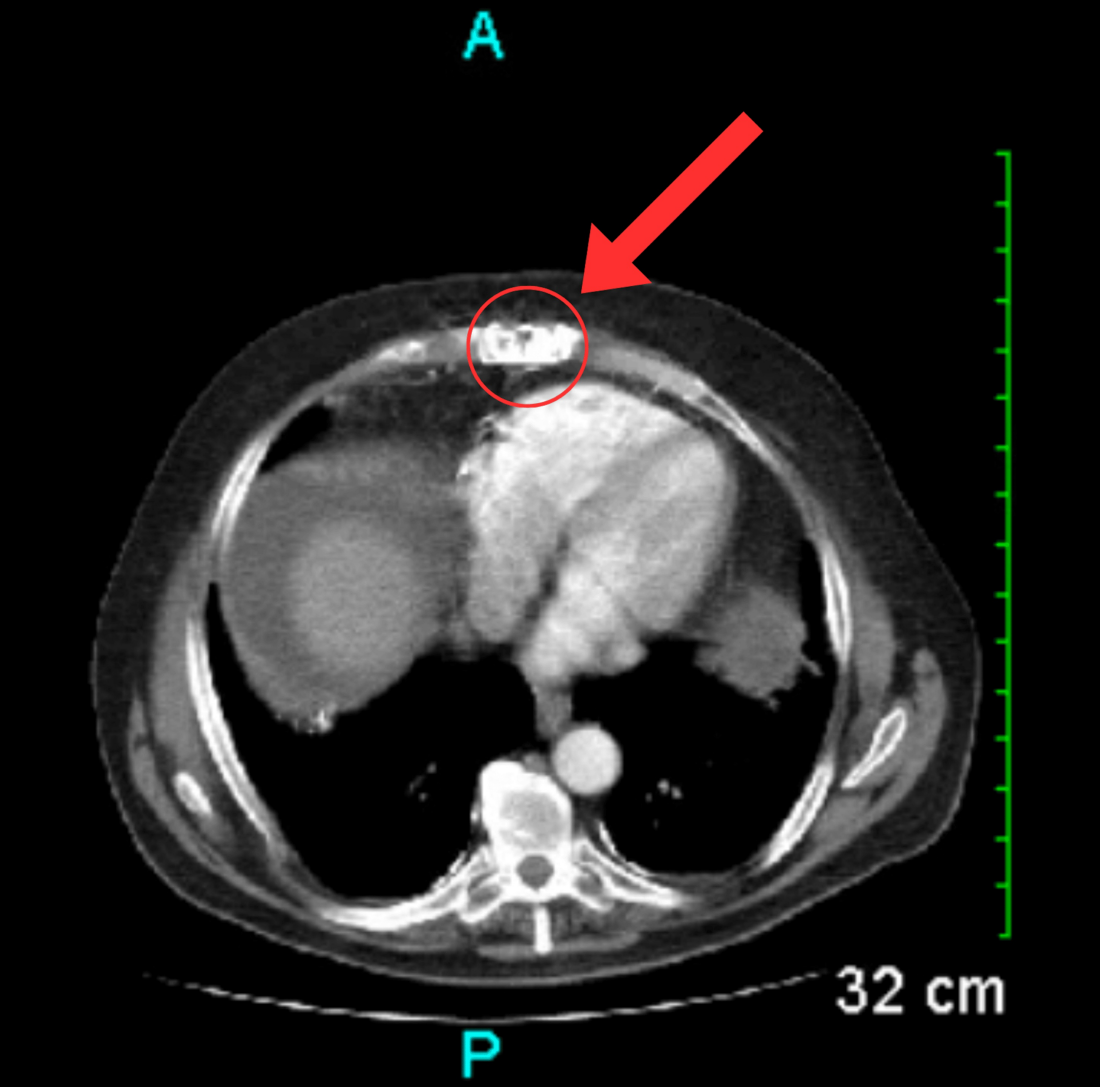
CT scan showing evidence of a prior sternotomy (red arrow and circle). CT: Computed tomography
